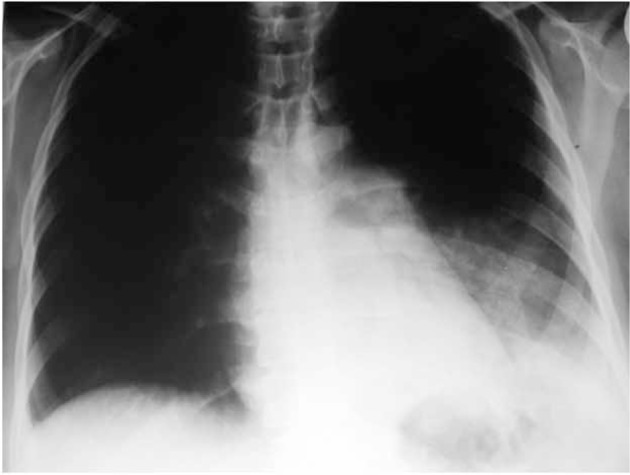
A 30-year-old female with respiratory symptoms and proved H1N1 infection. Chest x-ray demonstrates left pleural effusion. Pulmonary segment is also prominent.
